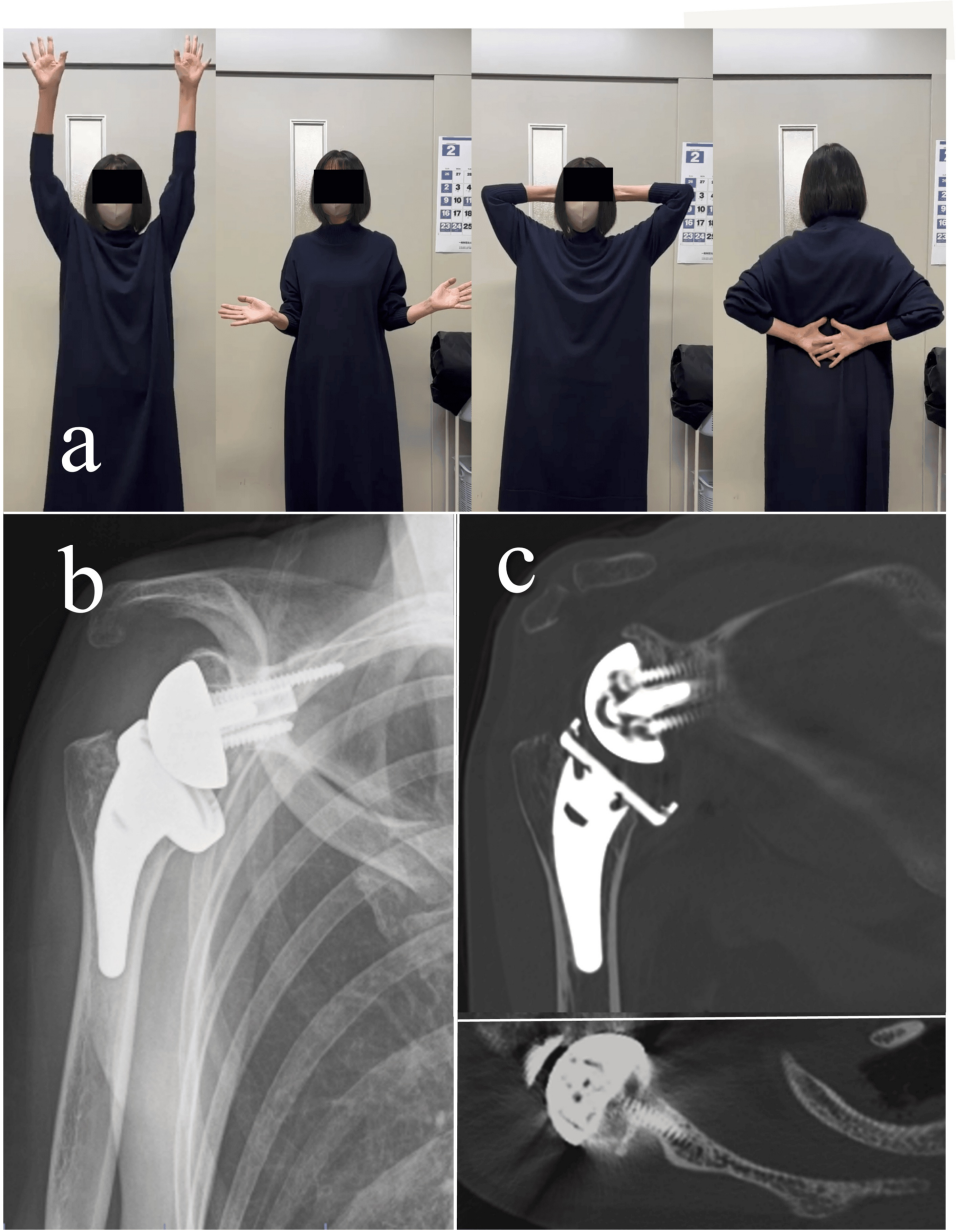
Postoperative course of the shoulder. (a) Shoulder range of motion two years postoperatively. (b) Plain radiograph immediately after surgery. (c) Computed tomography (coronal and axial views) two years postoperatively.
